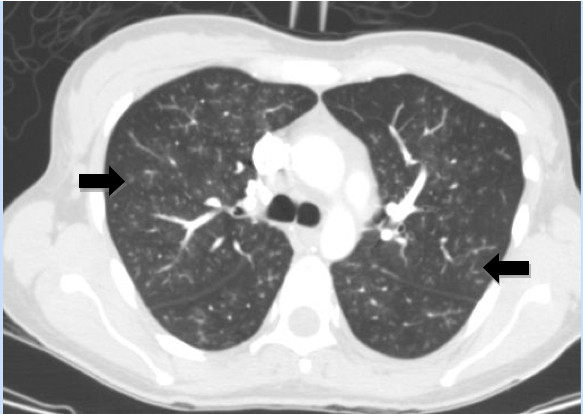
High resolution CT scan shows diffuse pulmonary involvement with ill-defined centrilobular nodules and branching lines (arrows), which correspond to tree-in-bud appearance.
